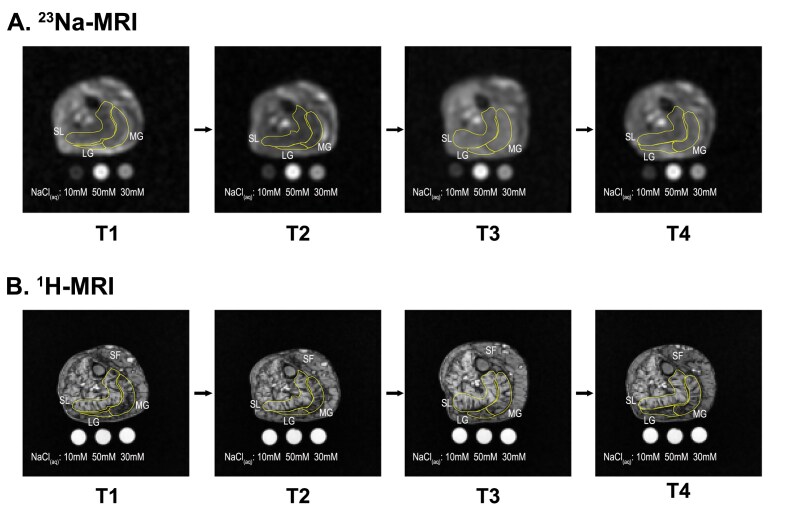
Representative (A) ^23^Na-MRI and (B) ^1^H-MRI images for timepoints T1–T4 obtained from the same patient. The calf muscle sodium concentration at each timepoint was derived by averaging the sodium concentrations in the three component muscles (outlined areas) which were quantified by referencing the ^23^Na signals in muscles to those in the 10, 30, and 50 mM NaCl standards: T1, 21.7 mM; T2, 13.8 mM; T3, 22.3 mM; T4, 19.5 mM. Muscle boundaries were defined on the ^1^H-MRI images and then applied to corresponding ^23^Na-MRI images. SL, soleus; MG, medial gastrocnemius; LG, lateral gastrocnemius; SF, subcutaneous fat; NaCl_(aq)_, sodium chloride aqueous solution.
